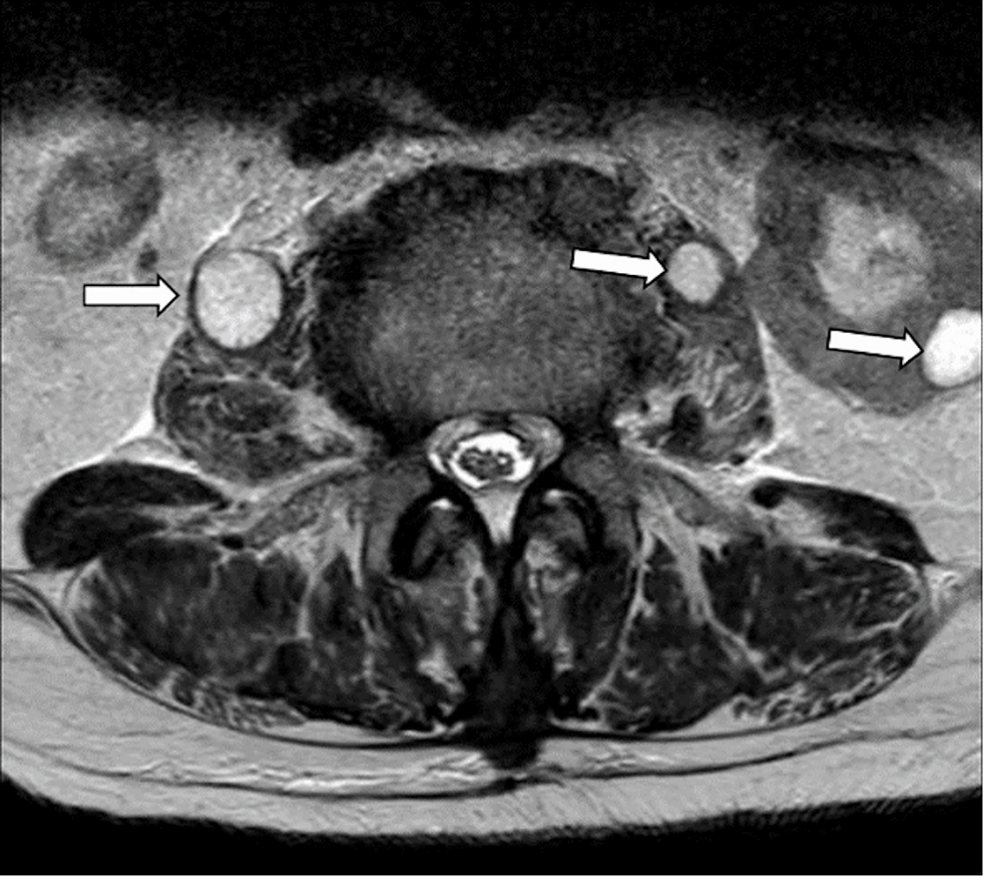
Lumbar MRI at presentation Transverse T2-weighted sequence highlighting cystic lesions in both psoas muscles and left kidney at level L2/L3.
